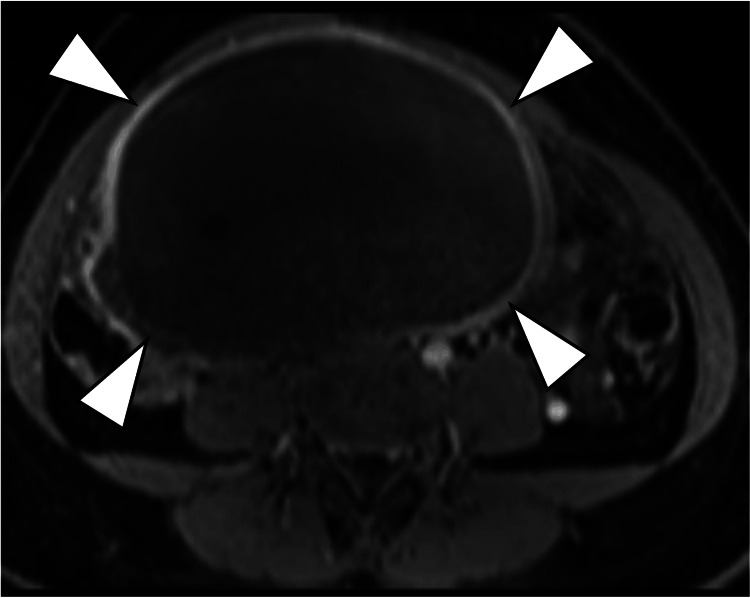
Axial, contrast-enhanced fat-suppressed T1-weighted Contrast-enhanced fat-suppressed T1-weighted image shows that only the capsular structures of the mass are enhanced (arrowheads).
